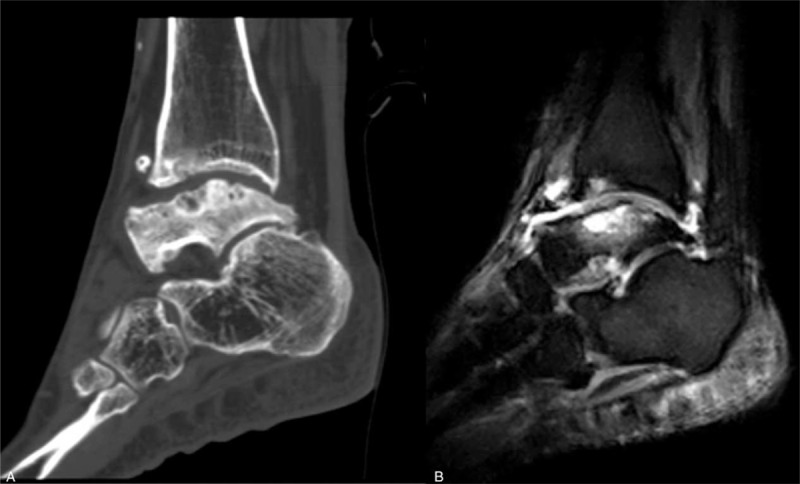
(A) The computerized tomography (CT) scan demonstrated that cystic lesions were developed at the dome of the talus and distal tibia. (B) Magnetic resonance imaging (MRI) showed extensive areas of high-signal intensity in the body of the talus and nonspecific inflammation in the left ankle and subtalar joint on T2-weighted images.
